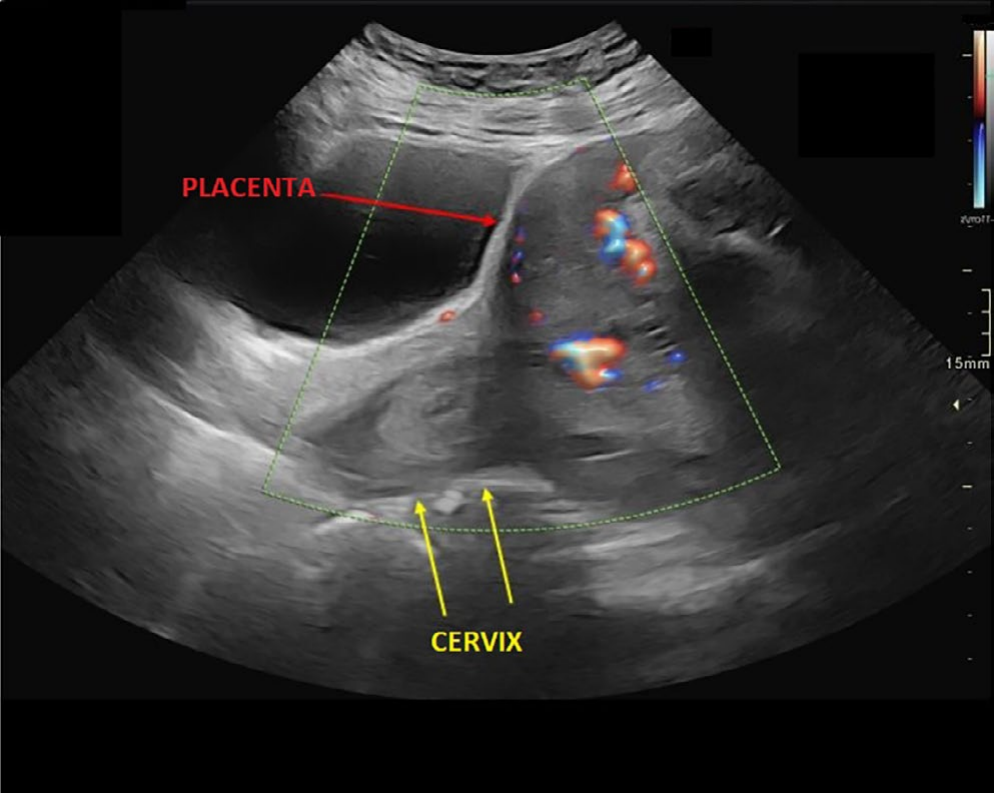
Ultrasound image showing the placenta previa and the cervix.
